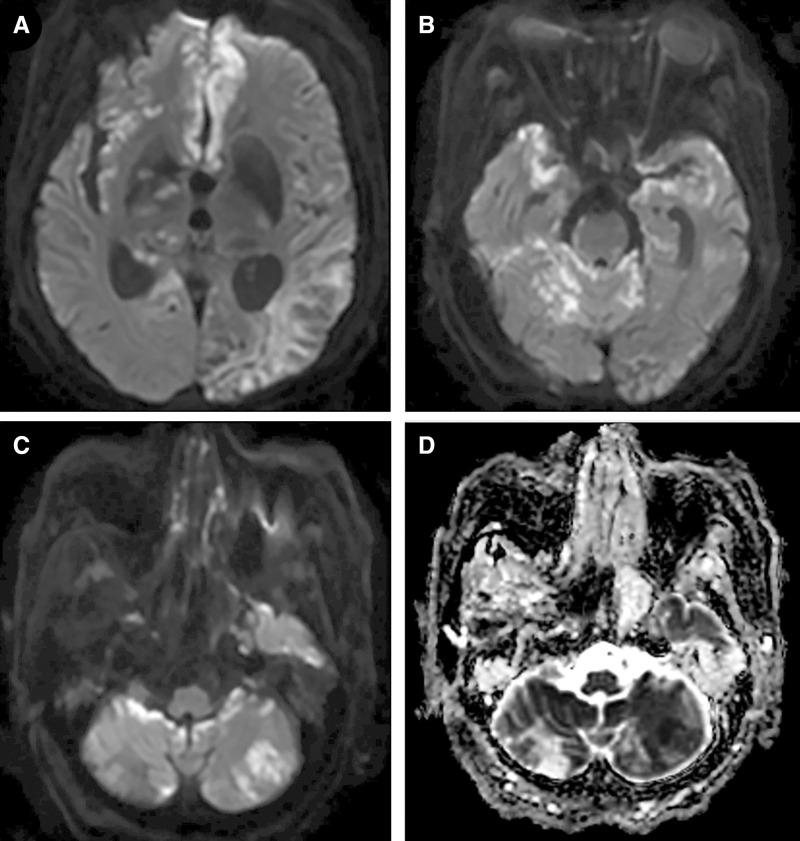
Brain diffusion magnetic resonance imaging. Axial diffusion-weighted image (DWI). (A) Hyperintensities in multiple lesions indicative of multifocal acute infarctions. High signal intensity along the right pons and in both cerebellum on axial DWI (B and C), along with slightly low signal intensity on the axial apparent diffusion coefficient map (D), suggest the possibility of meningitis.
